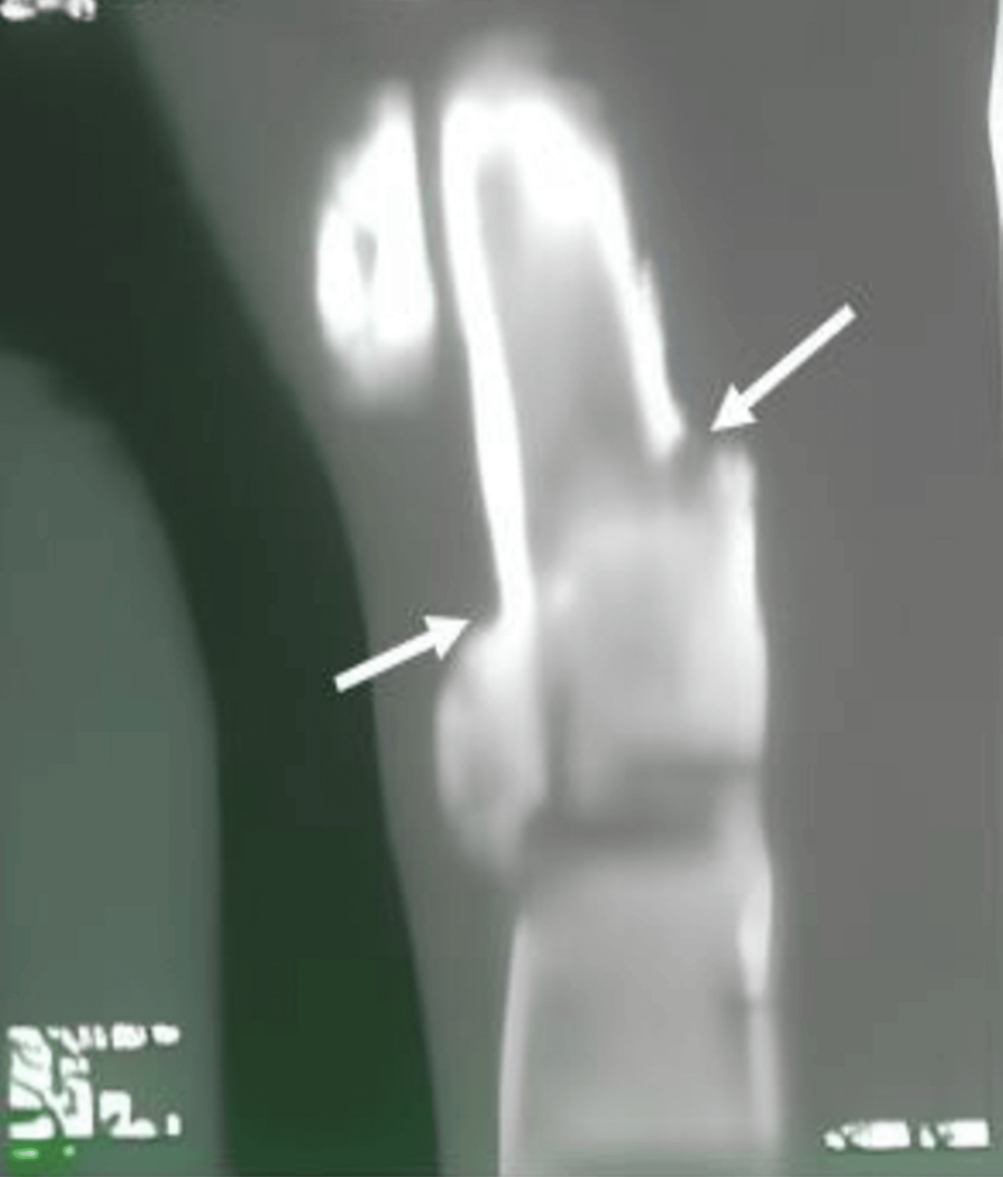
A CT shows a type II odontoid fracture in the second case (white arrows).
